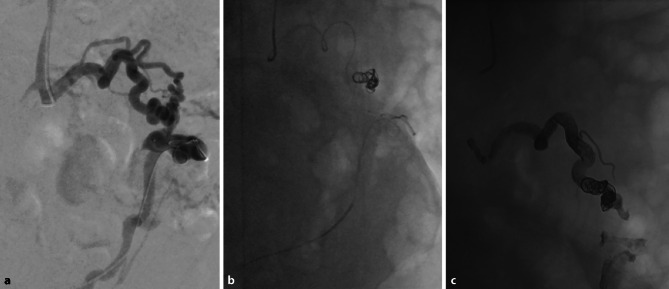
Tortuous veins forming a venous periumbilical convolute, as contrasted during interventional angiography. Catheters with coils in the proximal and distal segment of the convolute are shown (a). After successful selective embolisation from the transhepatic (upper end) and inferior epigastric (lower end) access, the detached coil is visible on x-ray (b). Onyx® embolus in situ with coil (c)
